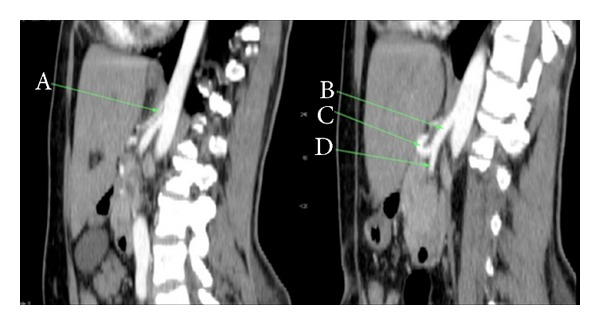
Sagittal reconstructed CT images demonstrating the new anatomic variant. Key: left gastric artery—A; unnamed common trunk—B; coeliac trunk—C; superior mesenteric artery—D.
